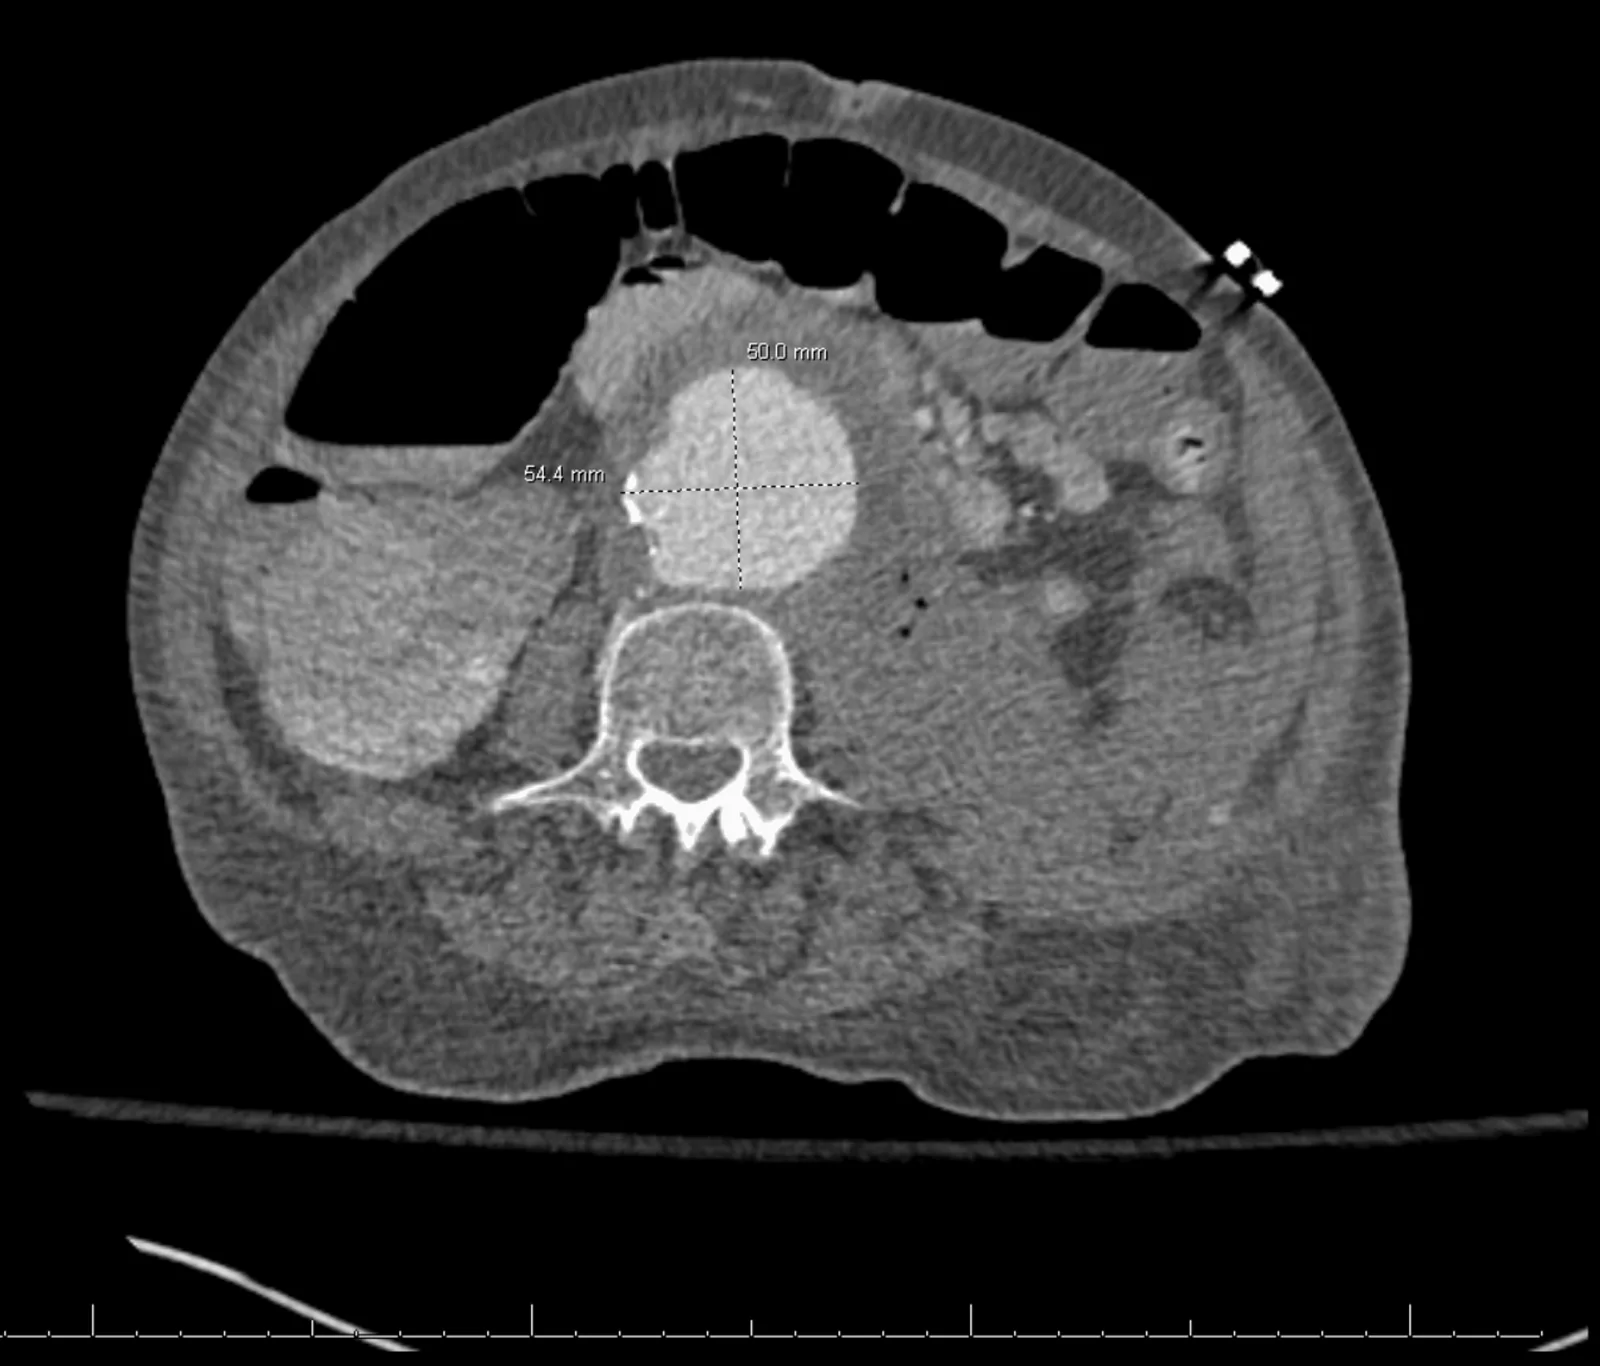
Axial computed tomography angiography (CTA) demonstrating the infrarenal abdominal aortic aneurysm. The aneurysm measures approximately 5.4 × 5.0 cm on the displayed axial image. CTA: Computed tomography angiography.
